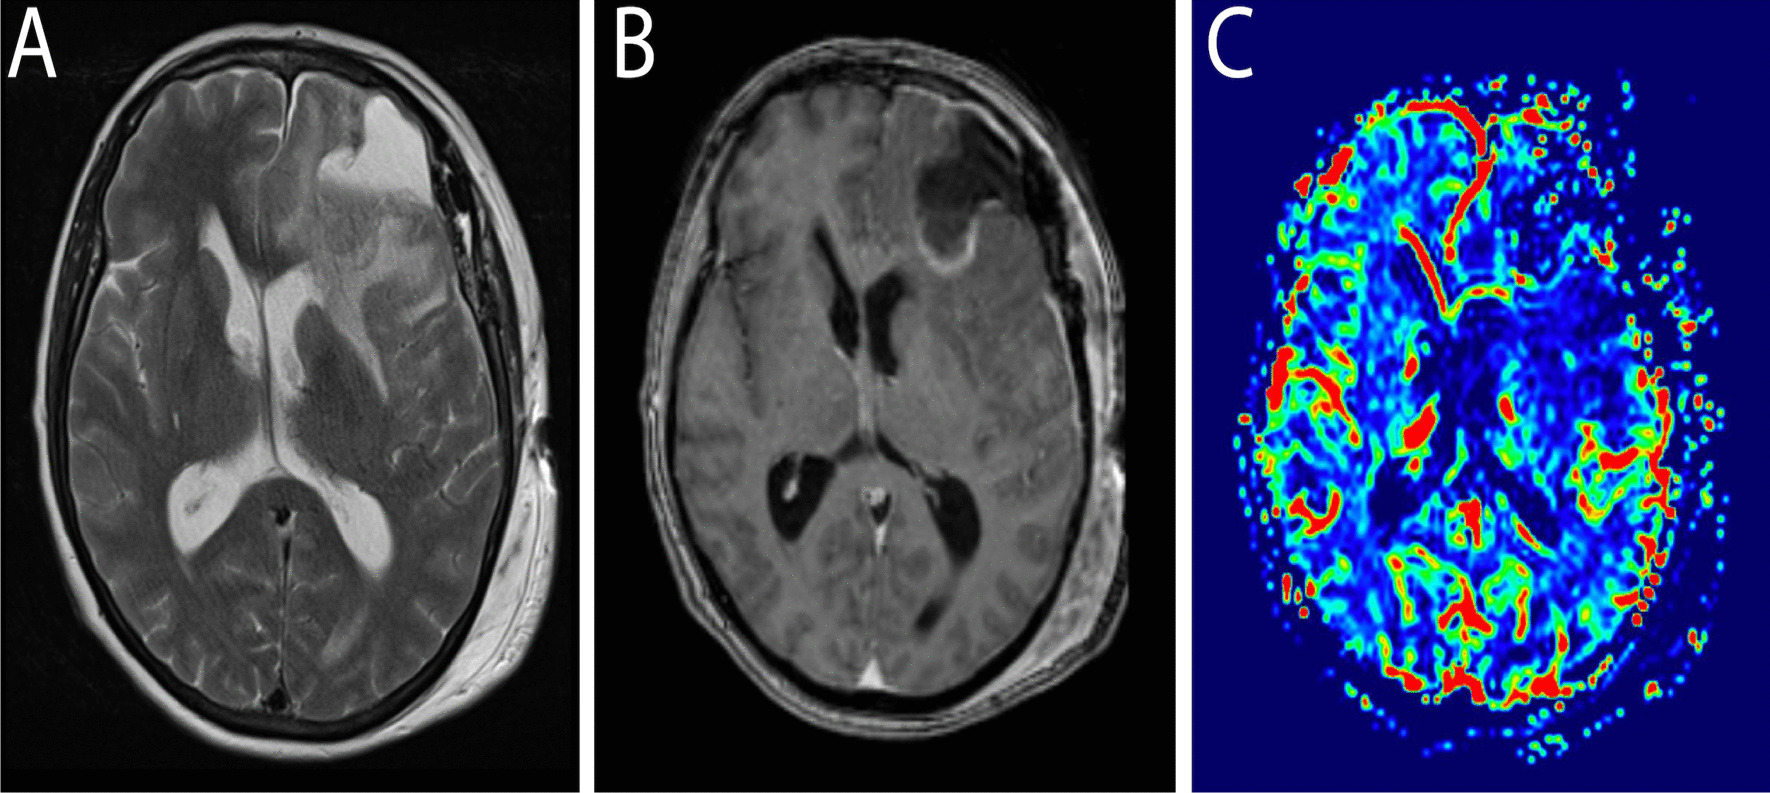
A Axial T2-w images show a reduction of the mass effect and persistence of surrounding vasogenic edema. B Axial postcontrast T1-w images show a reduction of the mass lesion. C Contrast-enhanced MRI perfusion imaging shows a reduction of high perfusion (green–red) areas
